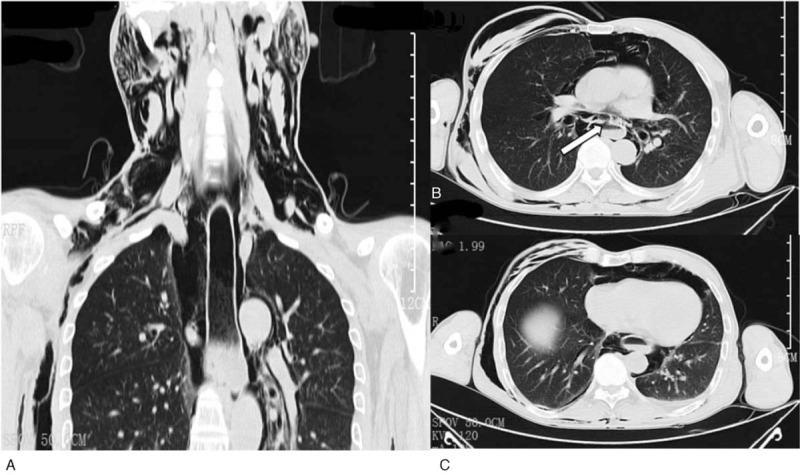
CT scan of the chest was showed extensive subcutaneous emphysema in the chest and back, a large amount of emphysema in the mediastinum, a small amount of left pleural effusion. (B arrowhead, Esophageal rupture). CT = computed tomography.
